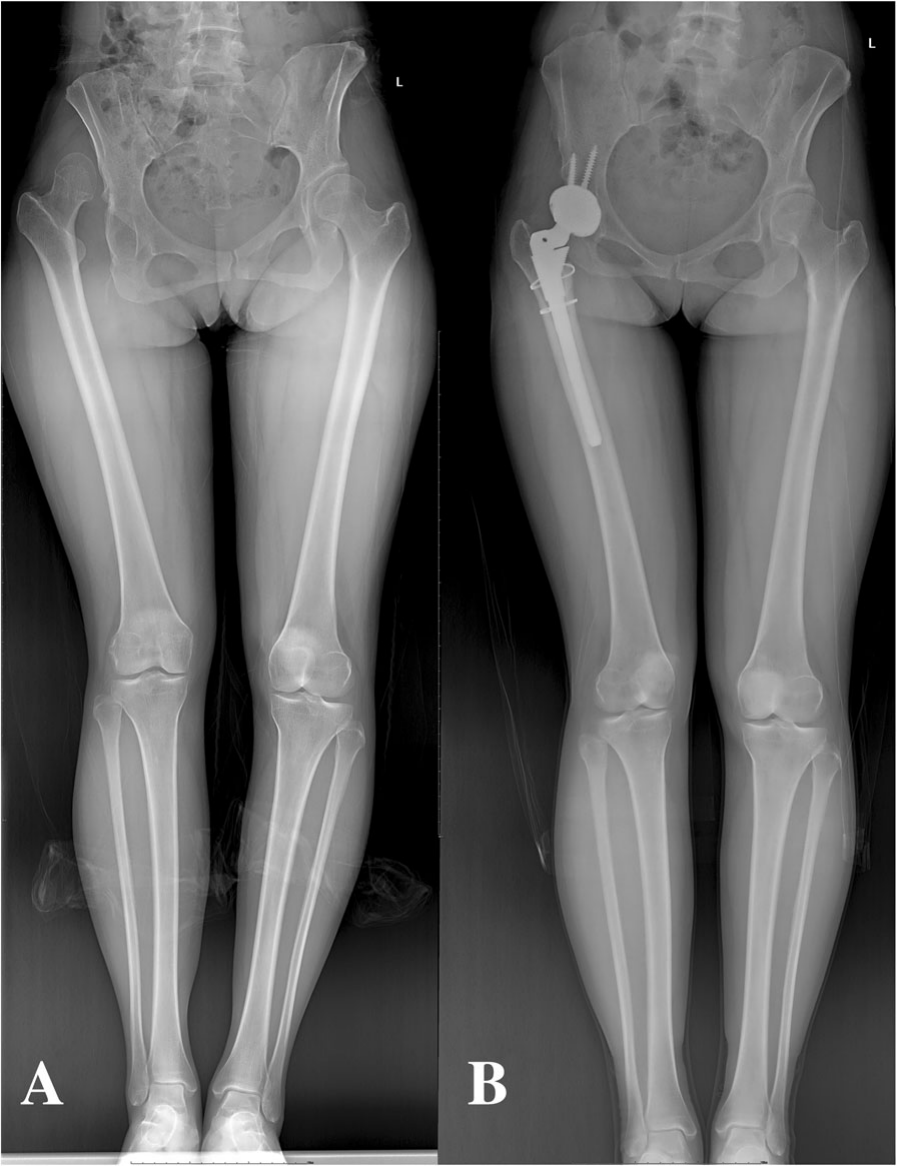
Full-length radiograph of lower limb of a Crowe-IV DDH patient. a The right limb is longer than the left limb preoperative. b The length of the affected limb is underestimated during the operation, and the length of the right limb was changed from shorter to longer postoperative
